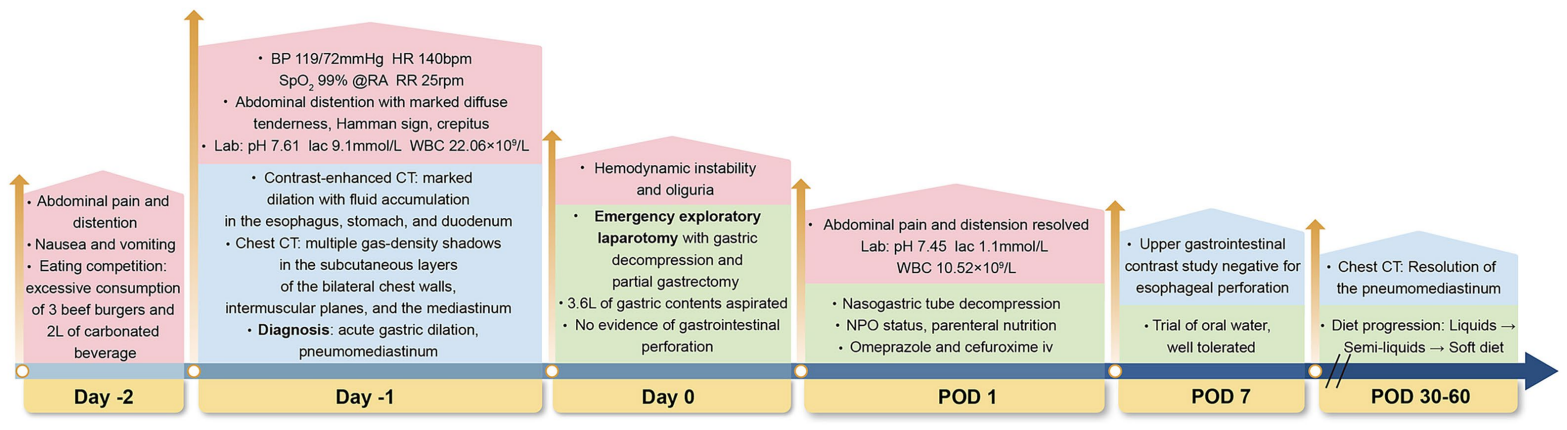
Timeline of diagnosis and management in a case of pneumomediastinum by acute gastric dilation after binge-eating. Day-2 (Symptom Onset); Day-1 (Hospital Admission); Day 0 (Emergency Surgery); POD, postoperative; BP, blood pressure; HR, heart rate; bpm, beats per minute; SpO_2_, oxygen saturation; RA, room air; RR, respiratory rate; rpm, respirations per minute; lac, lactate; WBC, white blood cell; CT, computed tomography pulmonary angiography; L, liter; iv, intravenous.
